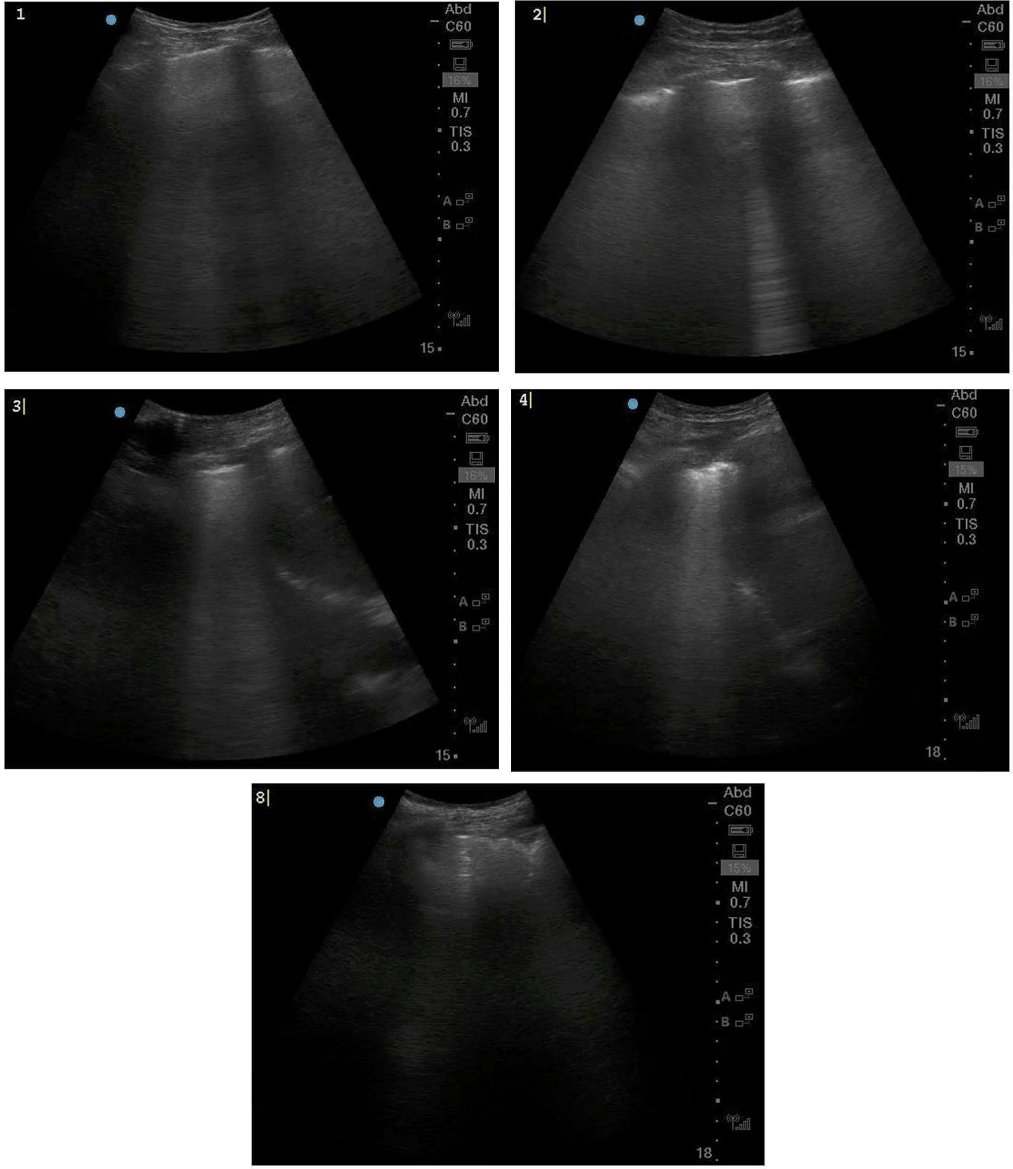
Point of care ultrasound shows B lines in lung zones 1, 2, 3, and 4 some areas of pleural irregularity, and subpleural consolidation in zone 8 (scanned in transverse orientation)
